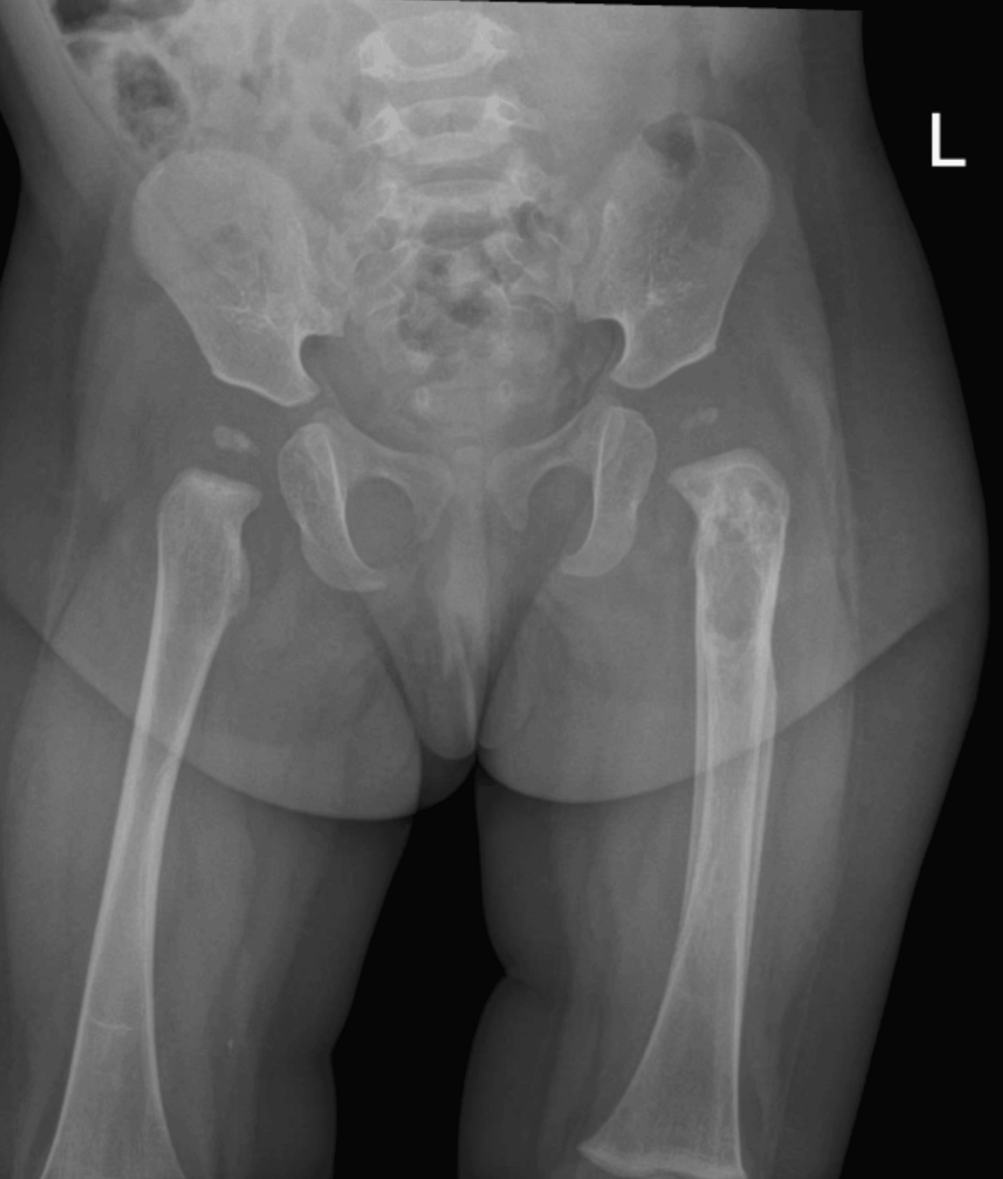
Follow-up X-ray of the pelvis including the midshaft of both femurs Follow-up X-ray of the left femur at six weeks showing new periosteal bone formation and sclerosis at the lesion site, indicating consolidation and early healing of the Langerhans cell histiocytosis lesion.
